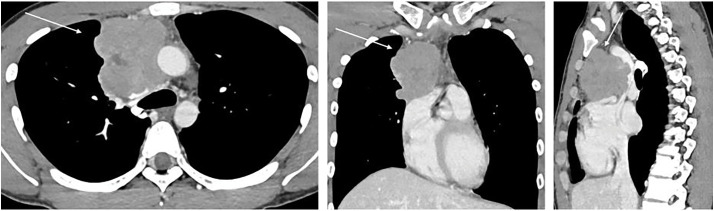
Chest computed tomography findings. An irregular mass measuring 80 mm was detected in the anterior mediastinum, with findings suggestive of invasion of the superior vena cava (arrow).
